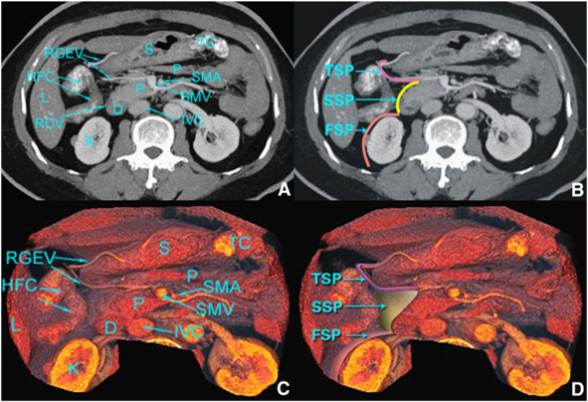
Following the administration of a dilute barium colon enema, MPR (a), MIP (b), and VR (c, d) are shown in the CT portal venous phase. The relevant anatomical features are labeled. *RGEV* right gastroepiploic vein, *FSP* first surgical plane, *HFC* hepatic flexure of colon, *SSP* second surgical plane, *L* liver, *RCV* right colic vein, *K* kidney, *TSP* third surgical plane, *S* stomach, *P* pancreas, *D* duodenum, *TC* transverse colon, *SMA* superior mesenteric artery, *SMV* superior mesenteric vein, *IVC* inferior vena cava
